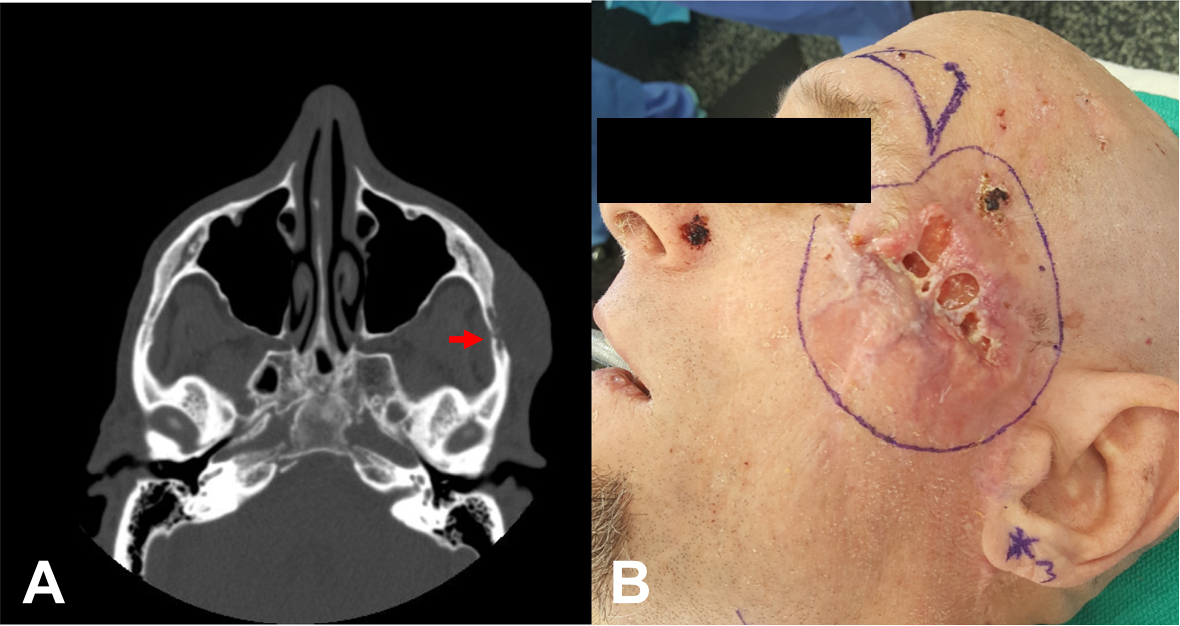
a Axial CT image revealing a 7 X 2.2 X 6 cm mass with extensive bony erosion of the left zygomatic arch (red arrow). b Preoperative digital image depicting a BCC that covers the full width of the left temple and a 6 mm BCC on the left nasal ala
